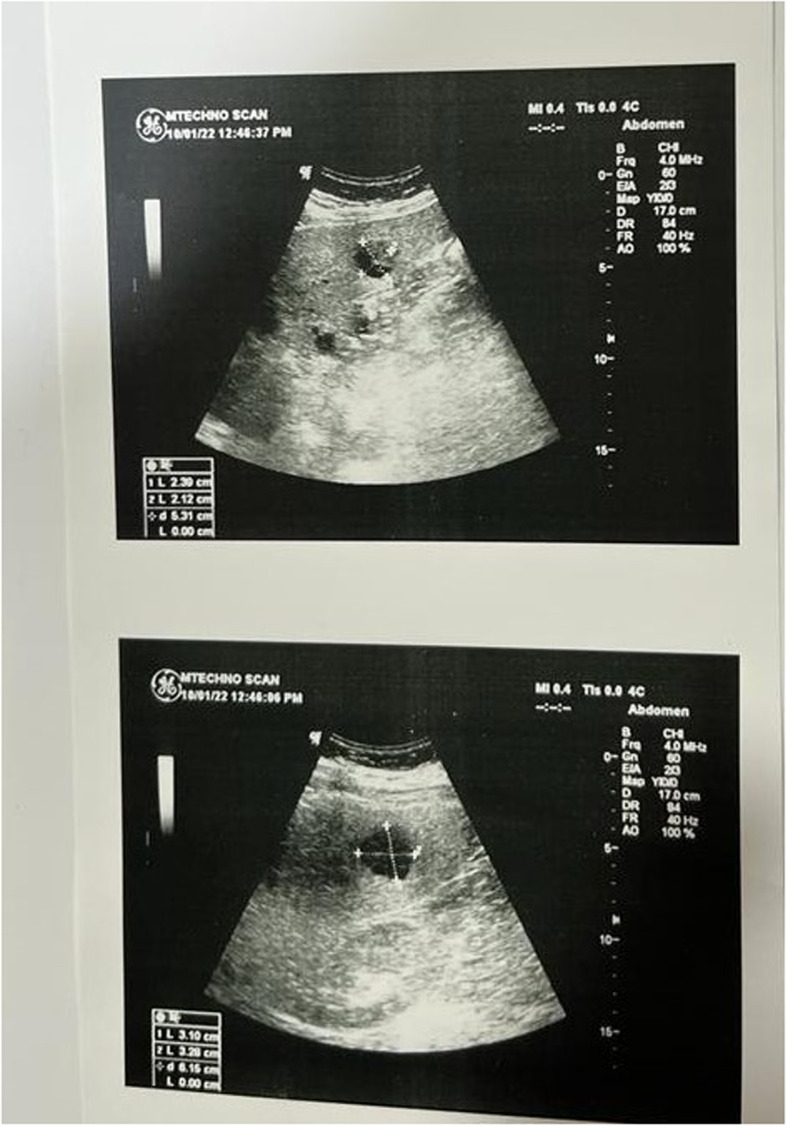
Abdominal ultrasonography. Multiple variable-sized hepatic complex cystic focal lesions
